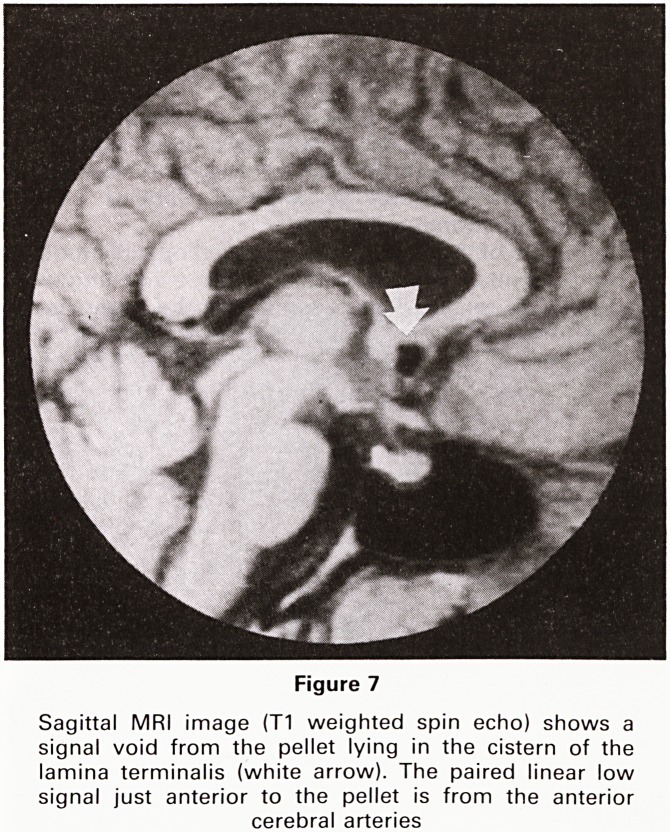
# Magnetic Resonance Imaging of the Orbit
